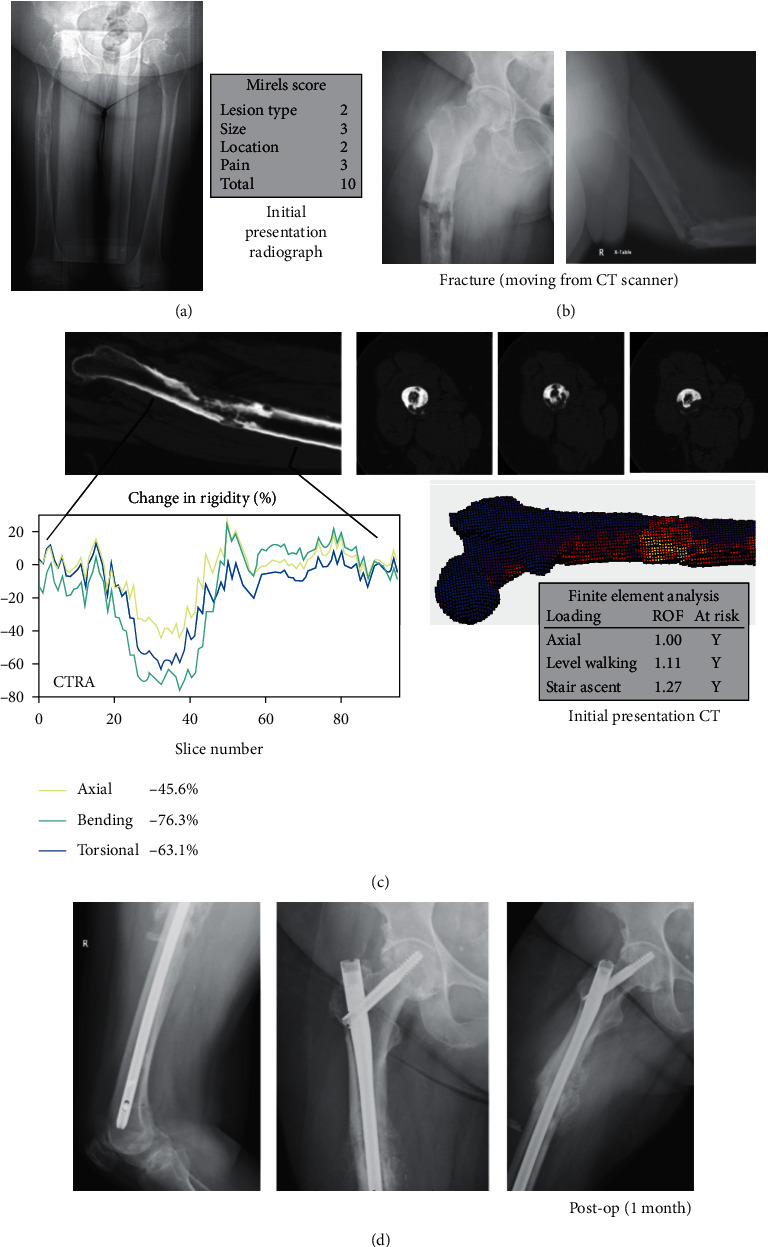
True positive (CTRA+/Fx+) (Case 1, R femur). (a) This is a 68-year-old female with metastatic breast cancer showing an osteolytic lesion in the right femoral diaphysis on X-ray imaging, (b) who, while moving from the CT scanner, fractured her right femur. (c) CTRA and FEA analysis both predicted fracture in this patient. (d) She was admitted and taken for surgical fixation of the right femur.
